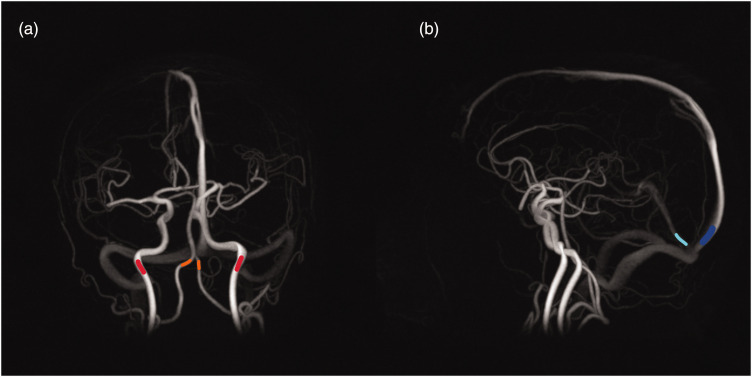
Location of flow measurements marked in angiograms from 4D flow MRI data. (a) Example of a location where arterial flow is measured. Internal carotid arteries are marked in red and vertebral arteries are marked in orange and (b) Example of a location where venous flow is measured. Superior sagittal sinus is marked in blue and straight sinus is marked in cyan.
